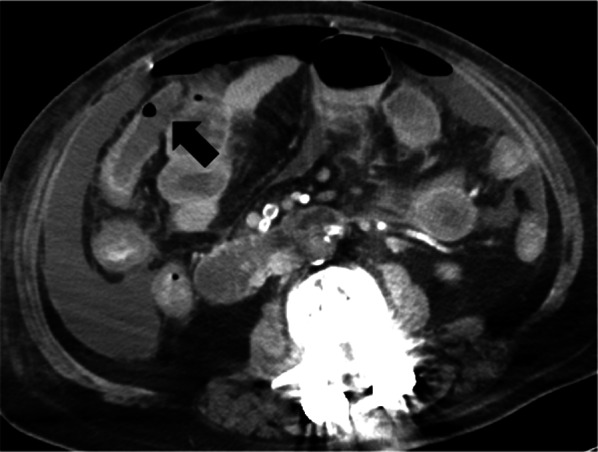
Computed tomography before emergency surgery showed massive ascites and free air as well as wall defects of the transverse colon (arrow)
